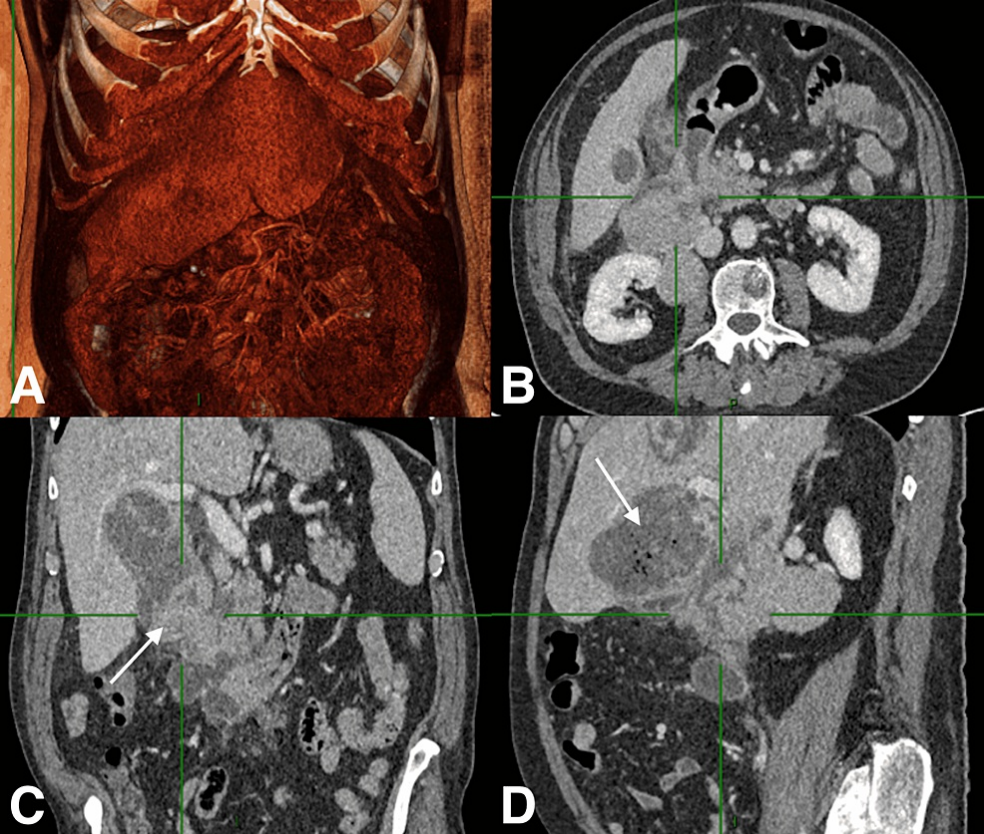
CECT with an invasive duodenal HCC nodule CECT, one week after the first TAE session, showed an invasive duodenal HCC nodule with persistent hypervascularization (white arrow in C) and tumor necrosis (white arrow in D) displayed with (A) VRT, (B) axial, (C) coronal and (D) sagittal planes. CECT: Contrast-enhanced CT; TAE: Transarterial embolization; HCC: Hepatocellular carcinoma; VRT: Volume rendering technique.
